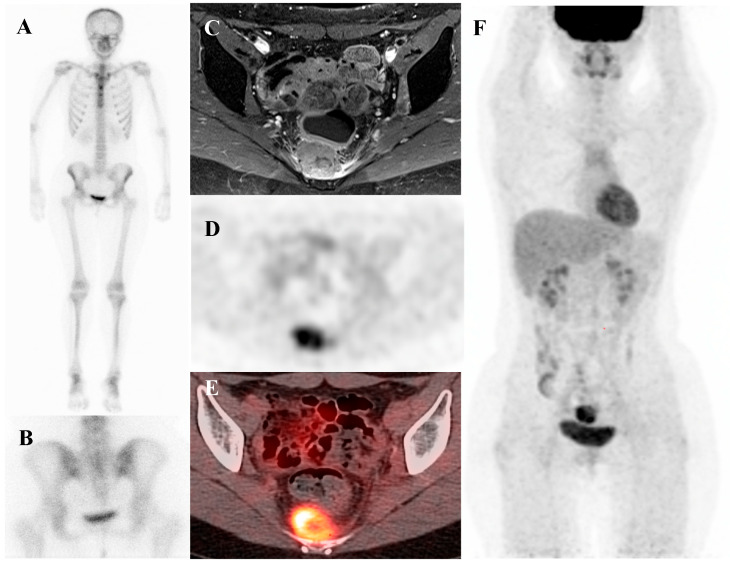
A 19-year-old female with recurrent Ewing’s sarcoma. (A,B) Bone scintigraphy shows no abnormal uptake on both anterior and posterior view. (C) T1 fat-suppressed magnetic resonance imaging demonstrates a lobulated, enhancing presacral mass with invasion of the sacrum. (D–F) [^18^F]FDG positron emission tomography/computed tomography reveals intense uptake of the mass (SUVmax 9.0). Surgical excision confirmed recurrence of Ewing’s sarcoma.
